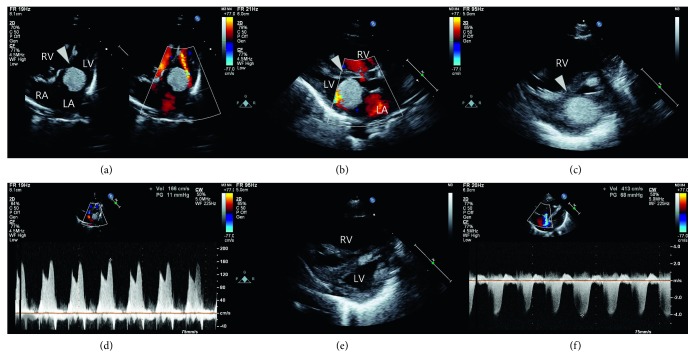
Two-dimensional echocardiography shows a large rhabdomyoma (arrowhead), 20.5 × 15.6 mm in diameter, occupying 75–90% of the mitral annulus, in addition to multiple small tubers in both ventricles and the left ventricular outflow tract after birth in apical 4-chamber (a), parasternal long-axis (b), and parasternal short-axis (c) views. Right atrial, right ventricular (RV), and left atrial dilatation were also observed. Pulse Doppler echocardiography showed a peak velocity through the mitral valve of 1.66 m/s (d). RV dilatation and pressure overload secondary to pulmonary hypertension were observed in a parasternal, end-diastolic short-axis view (e). The peak velocity through the tricuspid valve was 4.13 m/s (f).
